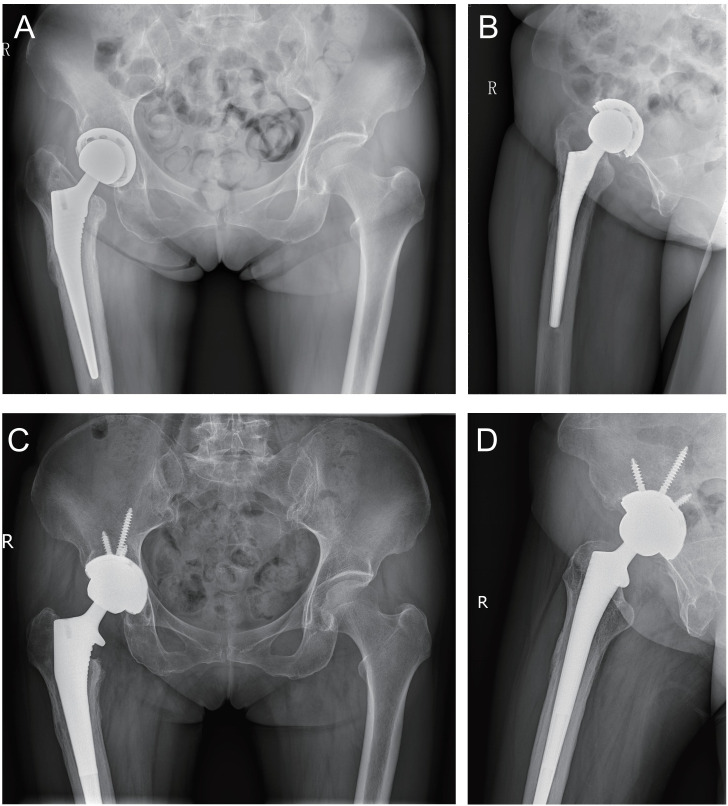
(A, B) represent the anteroposterior pelvic and lateral hip radiographs before revision surgery; (C, D) represent the anteroposterior pelvic and lateral hip radiographs 2 years after one-stage revision surgery.
